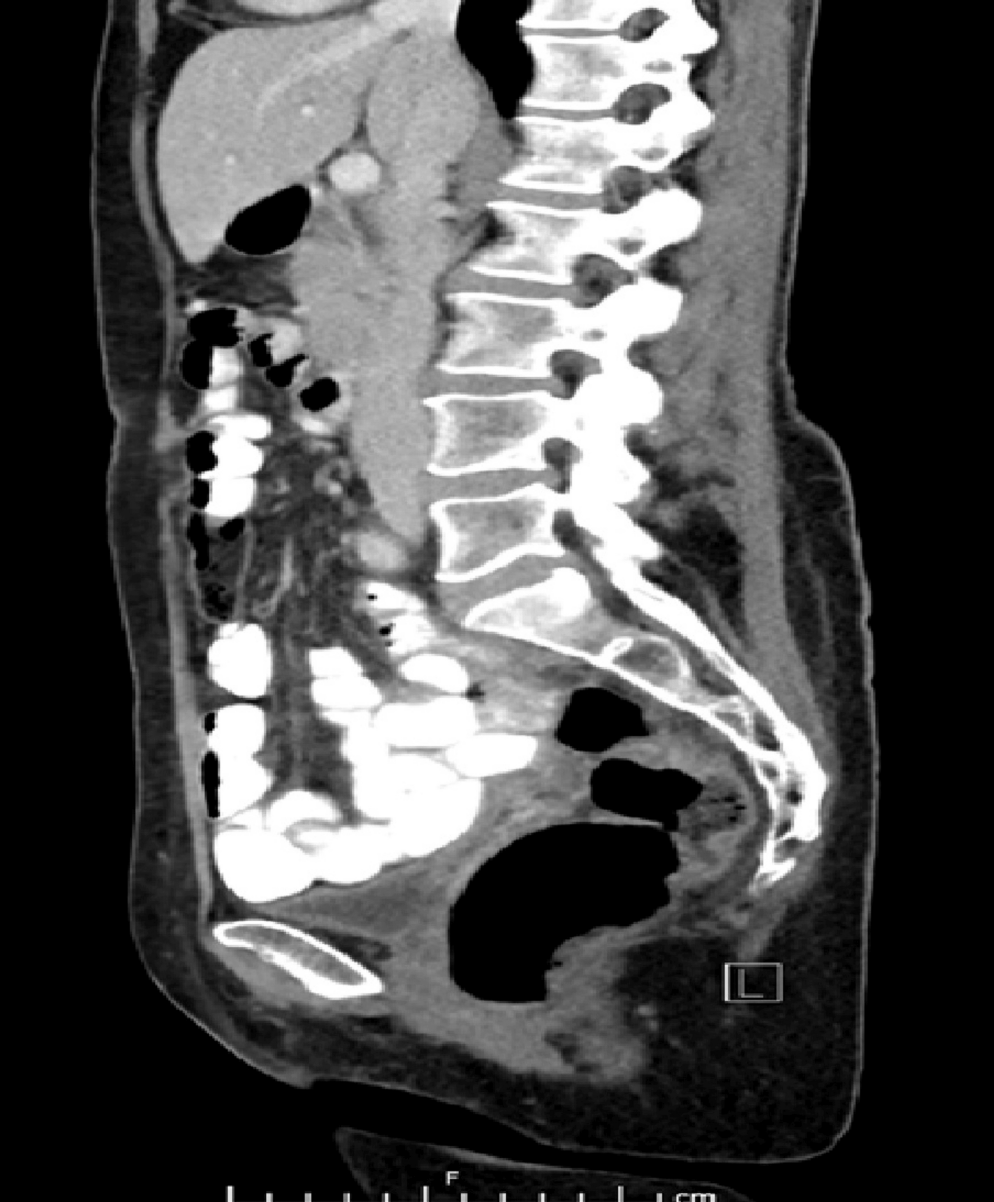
Midline sagittal cut of CT abdomen/pelvis
